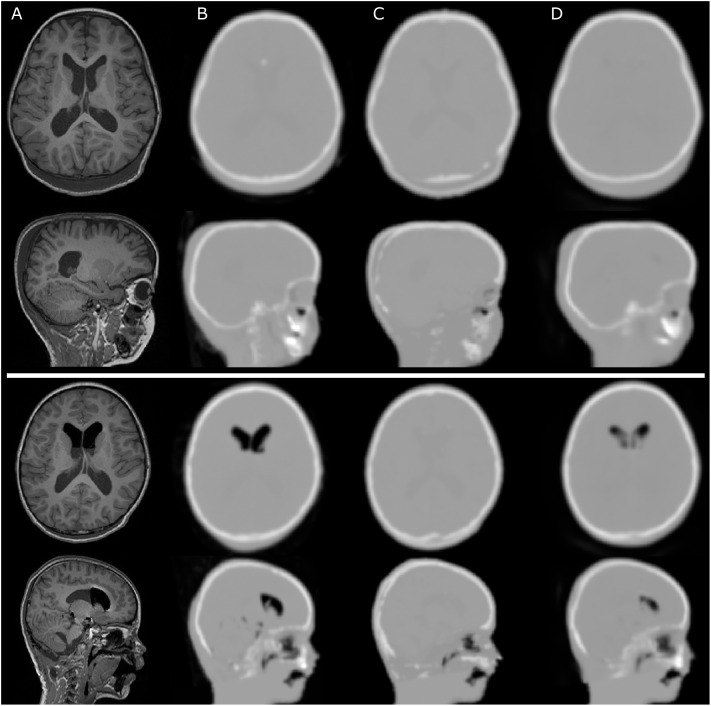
Sample cases for two pediatric patients with irregular anatomy. (A) show the T1w MPRAGE, (B) CT-AC, (C) RESOLUTE-AC, and (D) DeepUTE-AC. The top rows show a 5-year-old patient with post-operative subcutaneous soft tissue swelling in the occipital region. RESOLUTE erroneously fills in a dual layer bone layer on both sides of the swelling, along skin and bone. The bottom rows show a 6-year-old patient with air pockets anteriorly in the lateral ventricles that appeared after surgical intervention, and are not imaged in RESOLUTE. Also in this case RESOLUTE crafts a dual layered skull in the occipital region. For both patients, RESOLUTE is challenged in the definition of facial and skull base attenuation value. DeepUTE captures the morphology more confidently.
